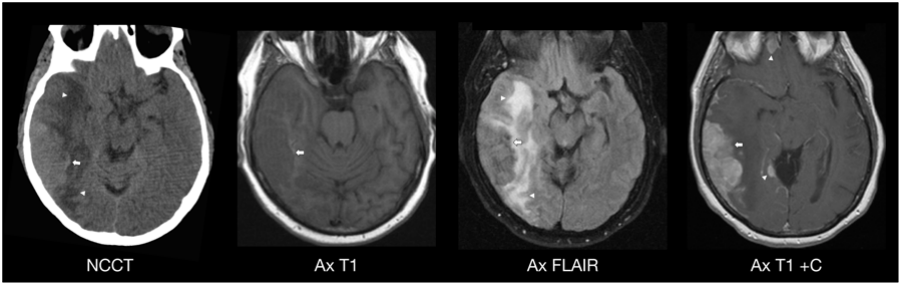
AML relapse in the form of a myeloid sarcoma mass lesion involving the right temporal lobe of a 56-year-old woman 39 days after umbilical cord blood stem cell transplantation. Noncontrast CT images demonstrate a large hyperdense dural-based mass lesion involving the right temporal lobe (NCCT, arrow) with surrounding edema (NCCT, arrowheads), mass effect and midline shift. On MRI, a large ill-defined avidly enhancing dural-based mass lesion involving the posterior aspect of the right temporal lobe (Ax T1 C+, arrow) with surrounding vasogenic edema (Ax FLAIR, arrowheads) was noted. This infiltrating mass revealed hypointense signal intensity on T1 (Ax T1, arrow) and T2-weighted images relative to the adjacent gray-matter. Diffusion weighted images (not shown) demonstrated restricted diffusion in the posterolateral aspect of the right temporal lobe mass consistent with increased cellularity. Additional pachymeningeal foci of nodular enhancement were noted within the gyrus rectus of the right inferior frontal lobe and within the medial aspect of the right inferior temporal lobe (Ax T1 C+, arrowheads). Diffuse right hemispheric pachymeningeal enhancement was also noted (Ax T1 C+, partially shown). Ax, axial; Sag, sagittal; FS, fat saturation; C+, post-contrast; DWI, diffusion-weighted images; ADC, apparent diffusion coefficient.
